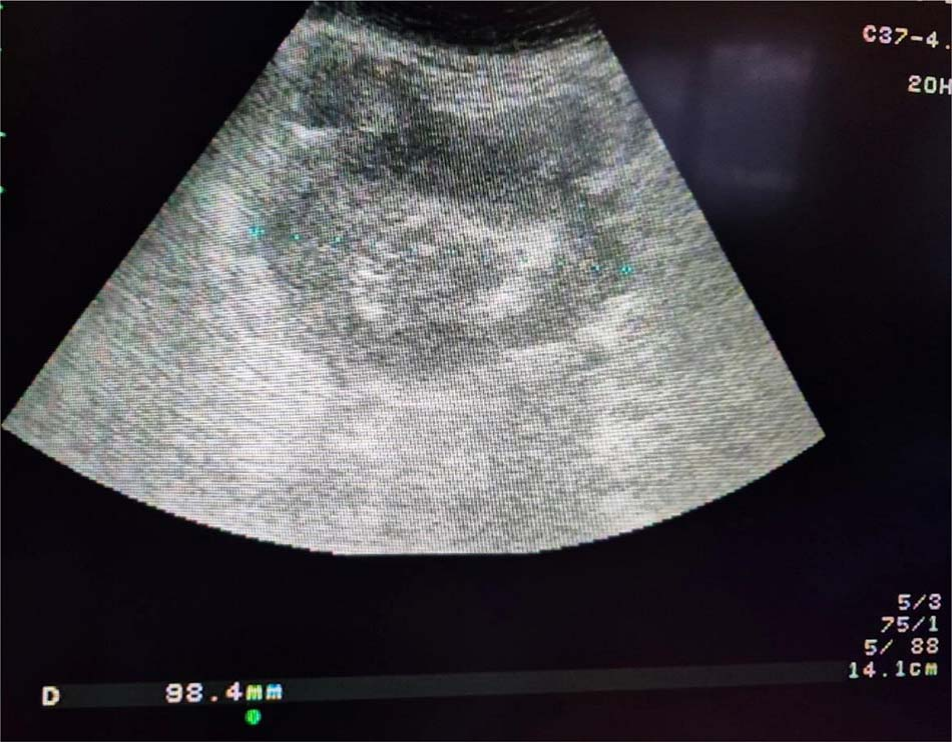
Ultrasound image of the small intestinal tumor.
